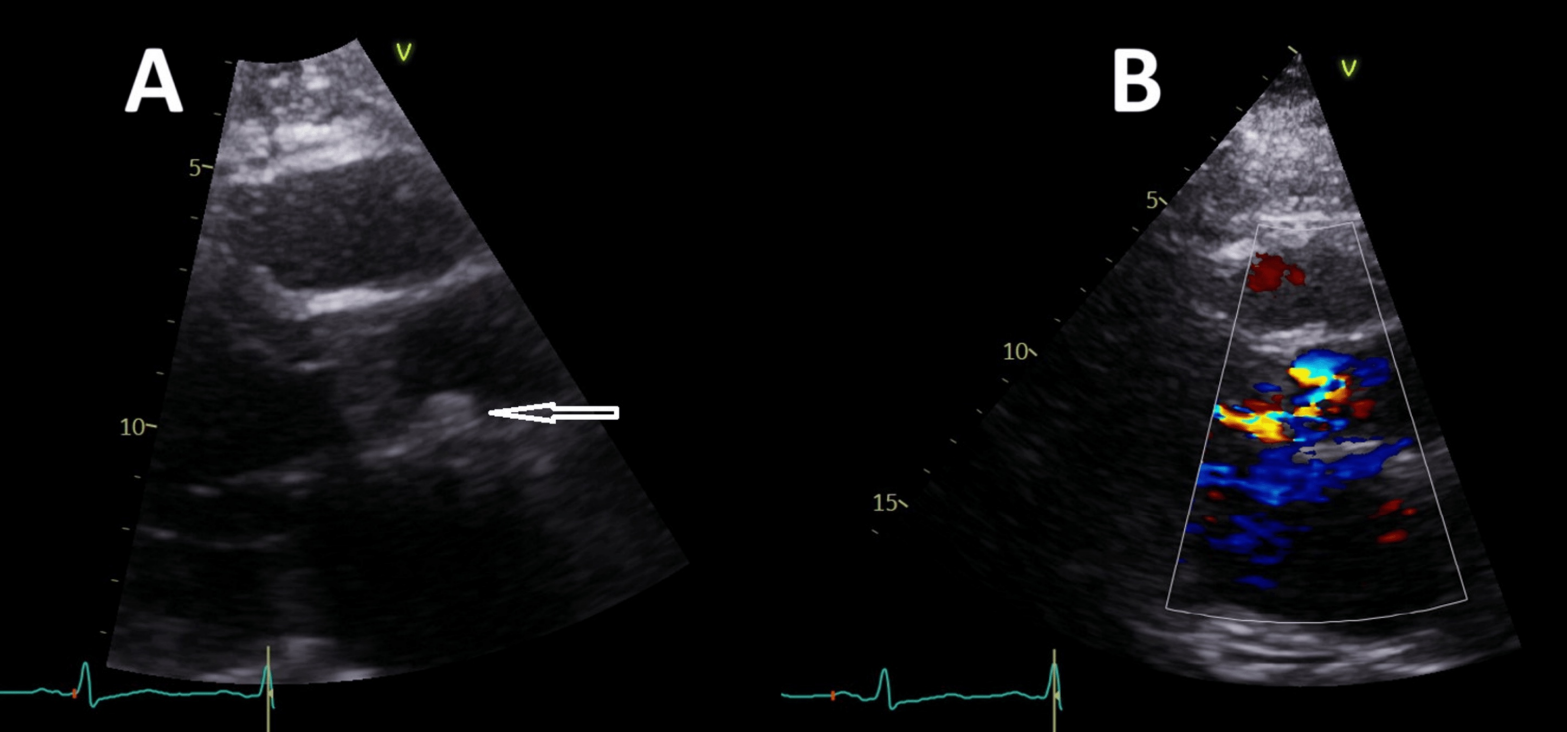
TTE showing (A) aortic valve vegetations (arrow) and (B) an aortic regurgitation jet. TTE: transthoracic echocardiogram
